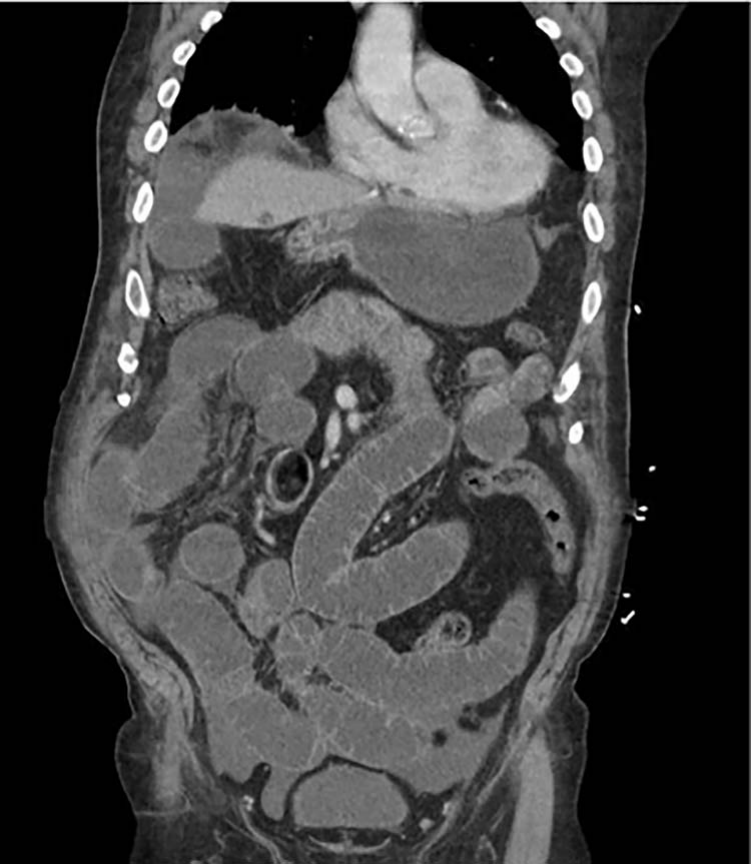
Abdominal CT scan on patient initial presentation.
